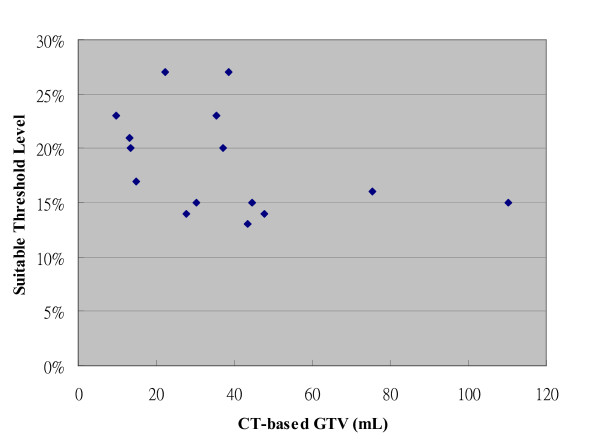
The association between the suitable threshold level and the CT-based GTV.
